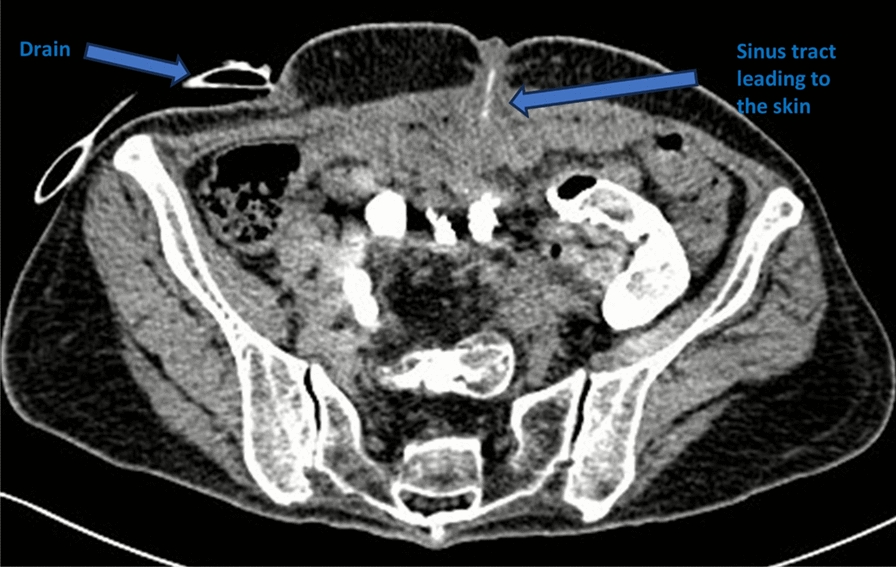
The computed tomography scan reveals an established enterocutaneous fistula (right-sided arrow) with an *in situ* drainage tube also visible (left-sided arrow)
